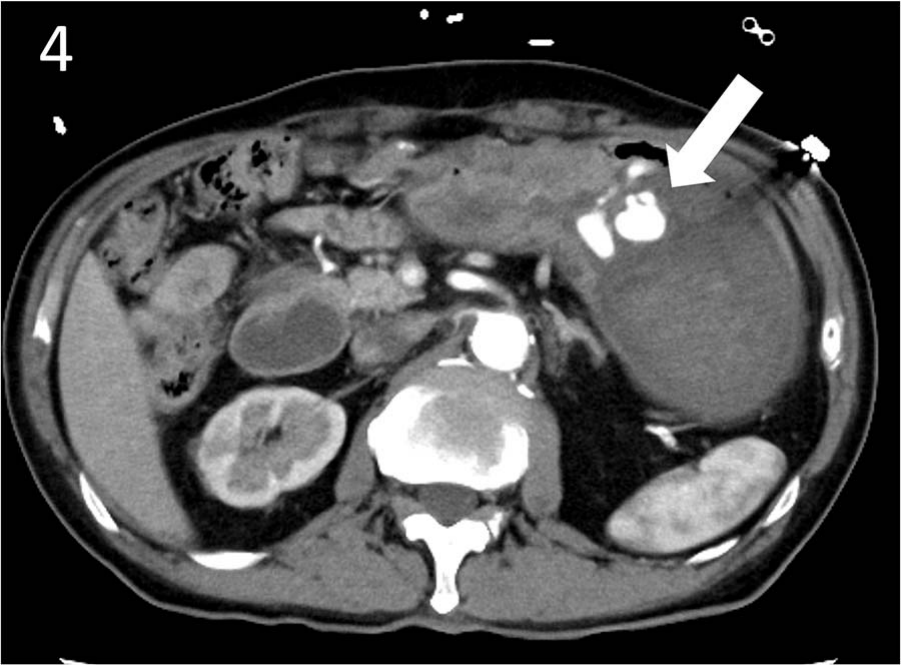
Contrast-enhanced computed tomography image showing active contrast extravasation in the stomach (arrow).
